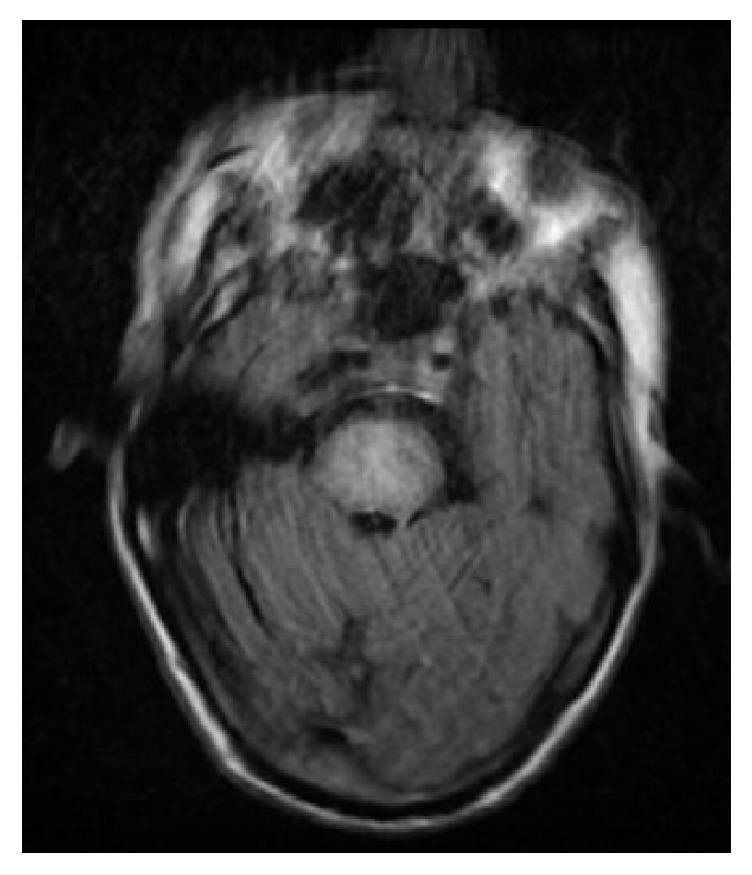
Magnetic Resonance Imaging of the brain without contrast showed high T2 signal and low T1 signal with restricted diffusion along with sparing of the bilateral peripheral pons, classic for central intrapontine, and extrapontine myelinolysis.
